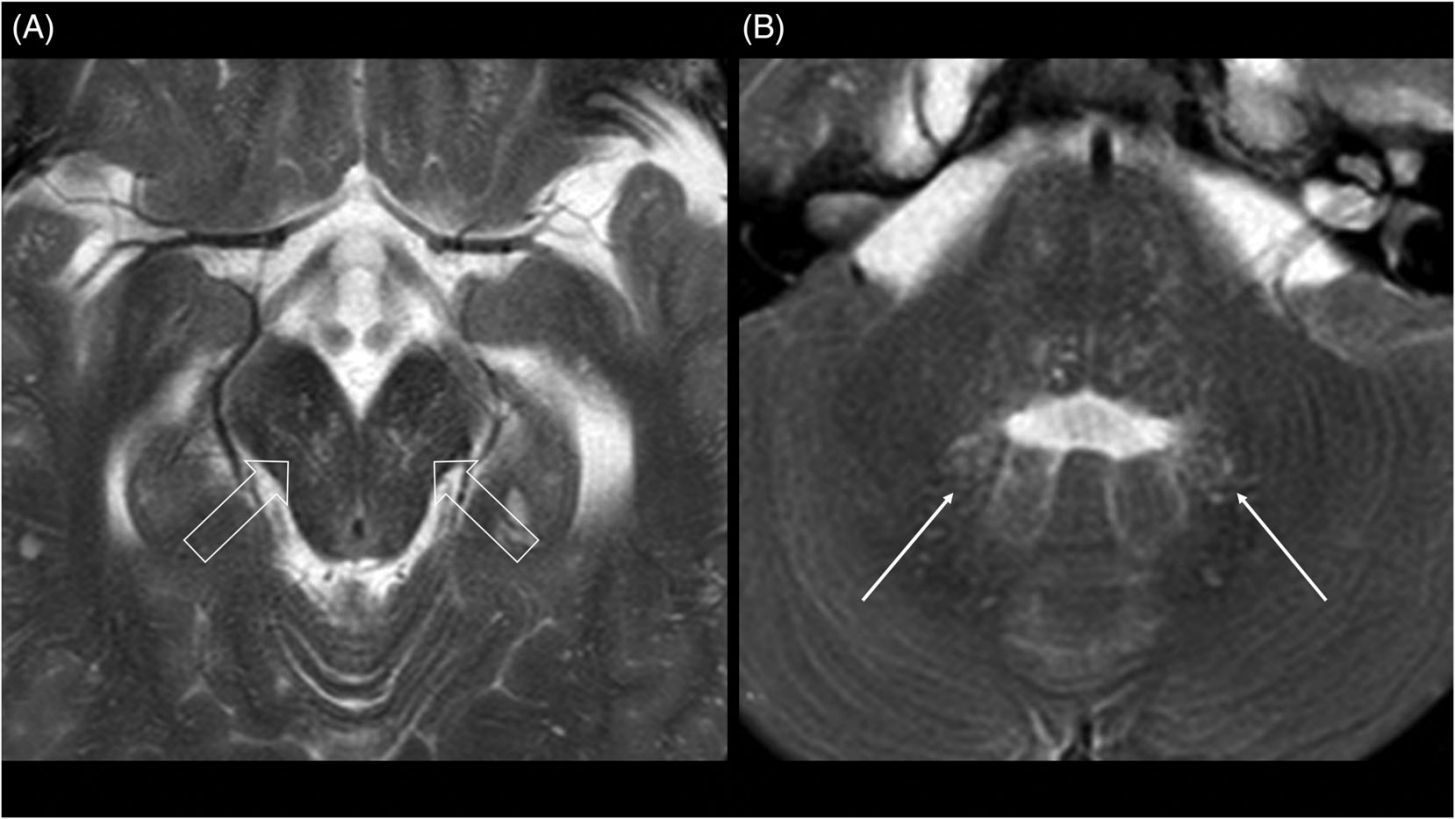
MPS IIB in a 26‐year‐old man patient. Axial T2‐weighted images show MRI‐visible perivascular spaces (A) in the cerebral peduncles (open arrows) and (B) in the white matter adjacent to the dentate nuclei (arrows). MPS, mucopolysaccharidoses; MRI, magnetic resonance imaging
